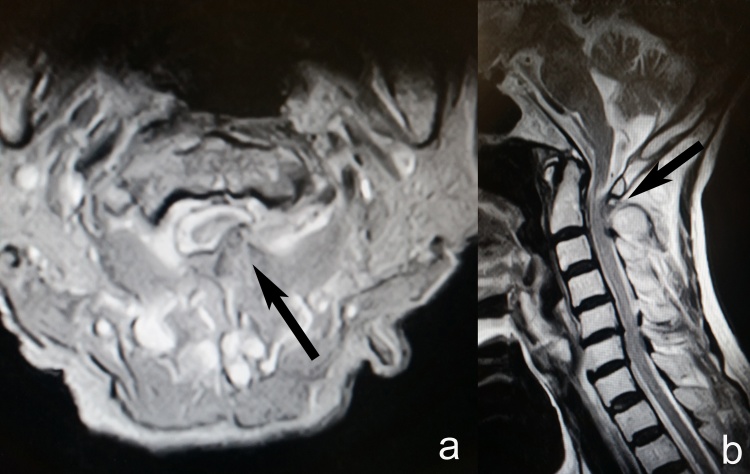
a) Cervical spine MRI showing the lesion (black arrow) on the left side, b) Cervical spine MRI showing the lesion arising from the left lamina of C2 vertebra, no other bony abnormalities noted.
